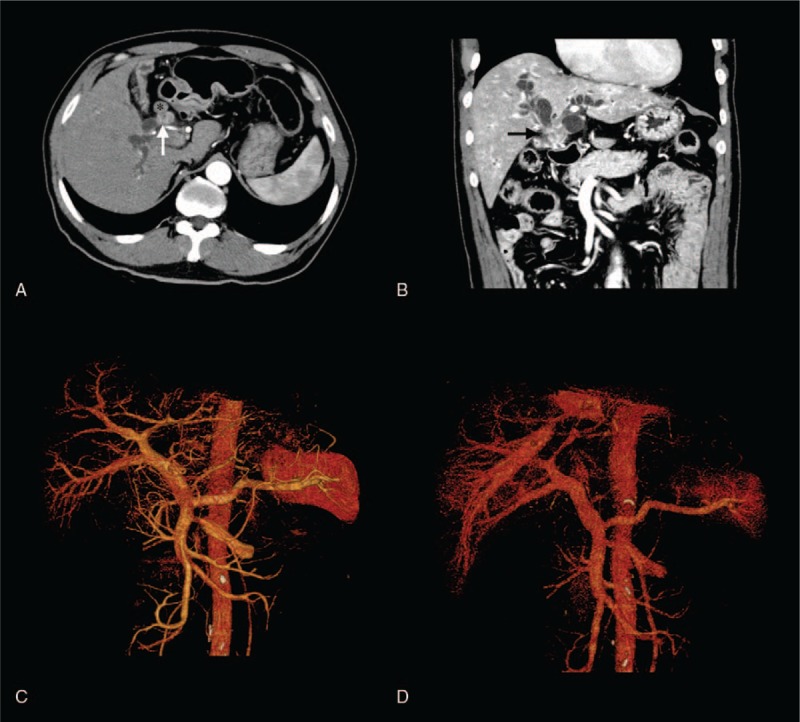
Abdominal enhanced computed tomography (CT) images. (A, B) An enhanced CT scan showed a moderately enhanced mass located in the hilar bile duct (white and black arrows) with regional lymph node involvement (asterisk). (C, D) CT angiography and 3-dimensional reconstruction revealed the absence of vascular invasion.
